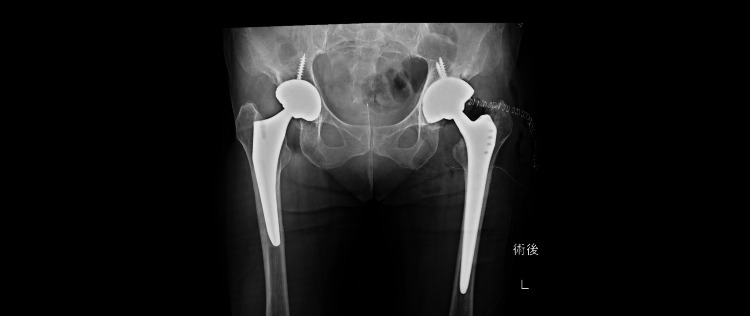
Hip radiograph from the two-stage revision surgery
